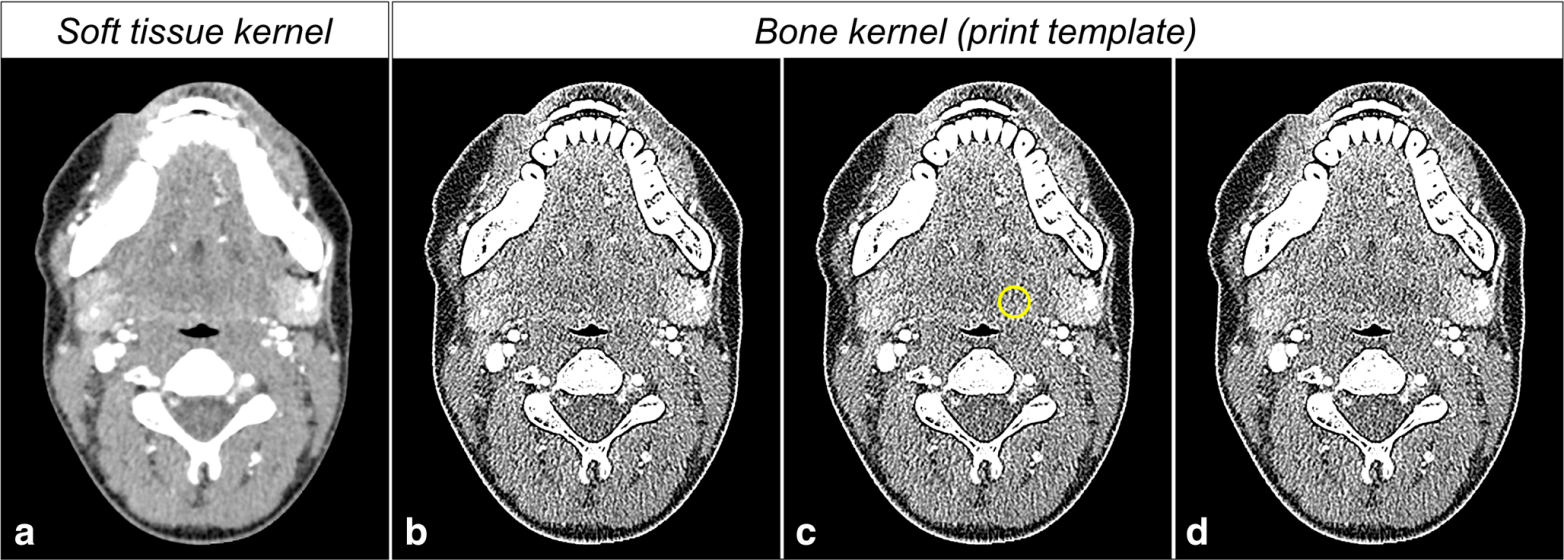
Manipulation of a CT image as a print template. a CT image of a patient (soft tissue kernel). b Bone kernel reconstruction of the same image. c Circular region of interest of 1 cm diameter in the left parapharyngeal space. d Manipulated image with a hypodense lesion of 40 HU contrast
